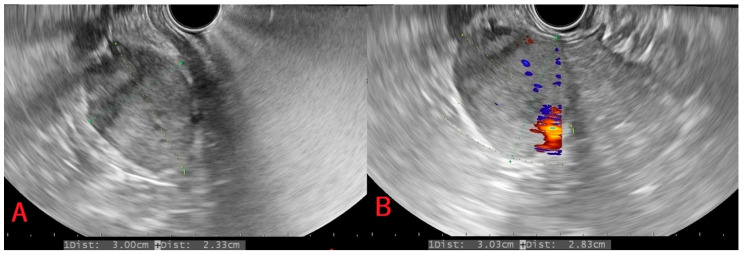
Case 2: Head of the pancreas tumor hypoechoic on ultrasound (A) with normal intensity Doppler signal (B).
